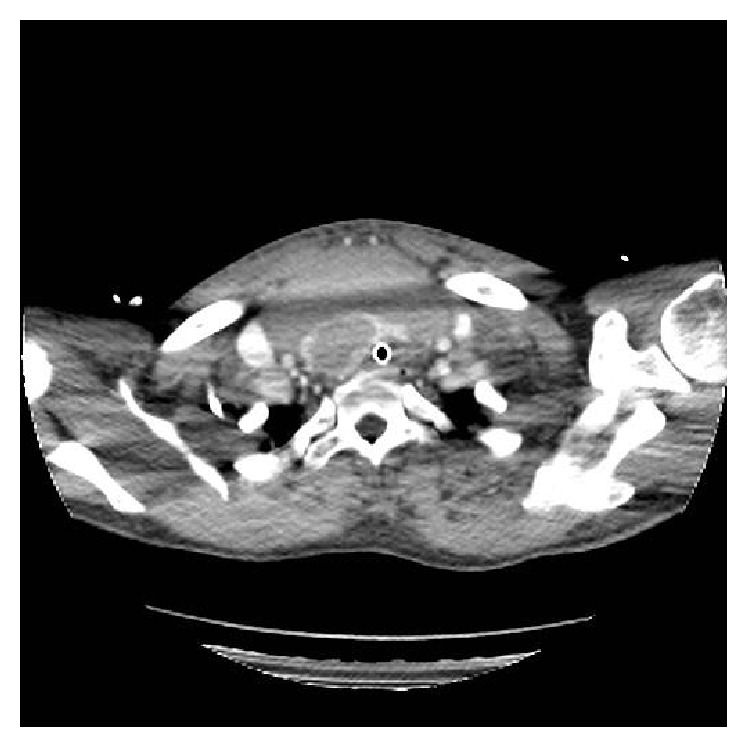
Arterial phase CT neck: axial view of neck haematoma demonstrating compression of the intubated trachea.
